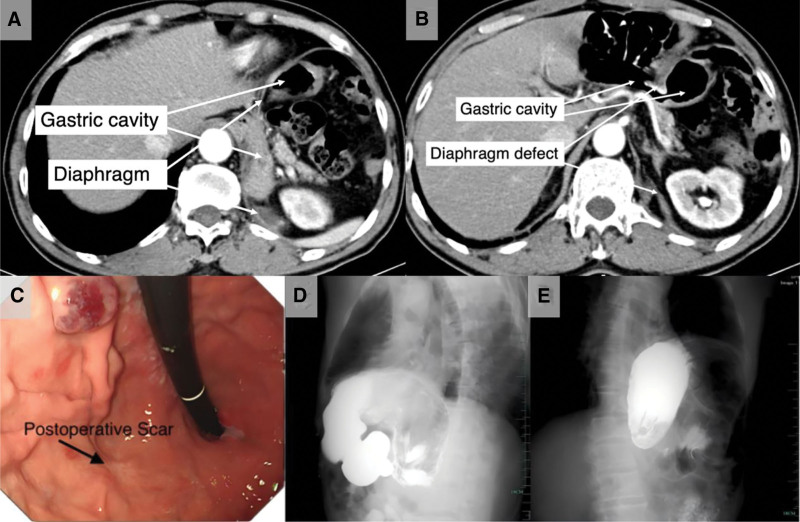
(A, B) Significant left hemidiaphragm elevation and defect. The herniated organs include partial stomach, small intestine, colon, spleen, kidney, and pancreas. (C) Gastroscopy revealed postoperative scar in the fundus, and this could be the location of the operation injury to the diaphragm. (D, E) Upper gastrointestinal radiography revealed a tortuous gastric cavity in the left thoracic cavity.
